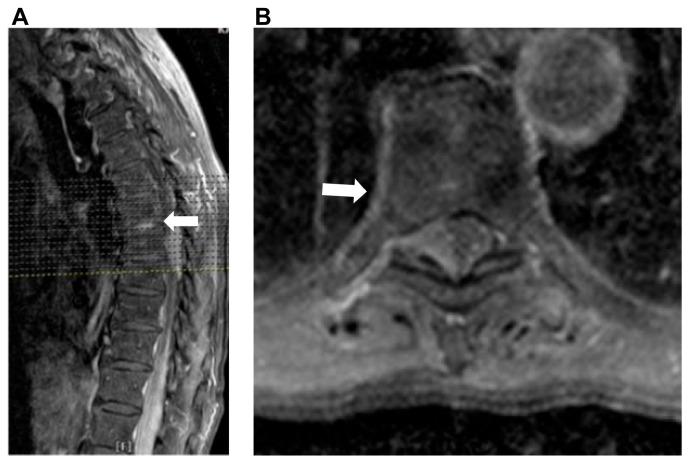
MRI of thoracic spine: (A) sagittal contrast-enhanced image showing a well-defined epidural soft tissue mass at the T7-T9 level (arrow); (B) axial contrast-enhanced image at the T8 level showing a right-sided, homogenously enhanced extradural lesion with spinal cord compression (arrow).
